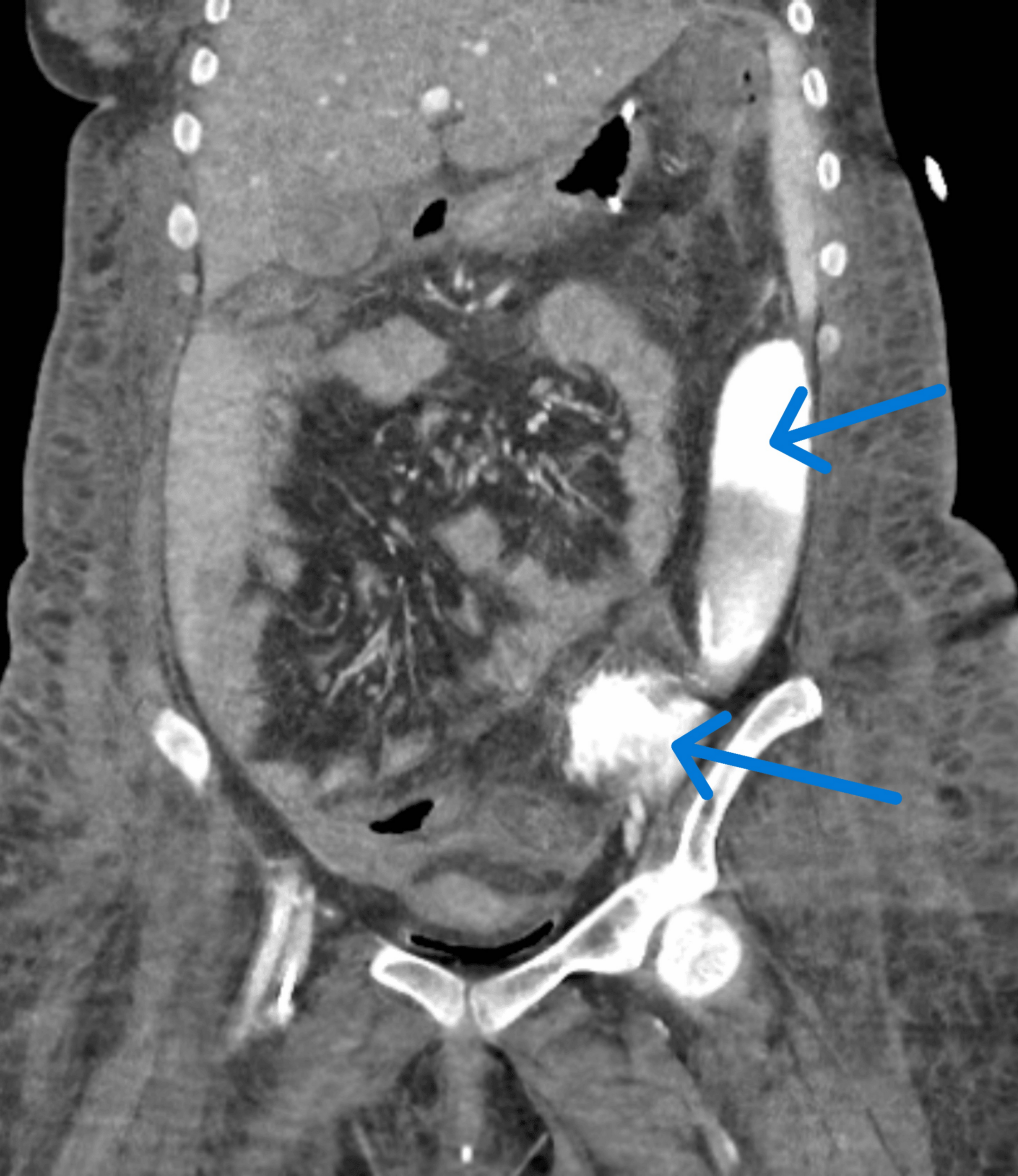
Contrast-enhanced CT of the abdomen and pelvis suspicious for a left distal ureteric calculus, resulting in the rupture/perforation of the left ureter in the left iliac fossa with resulting urinoma in the left retroperitoneal space, eventually extending into the peritoneal space in the left iliac fossa.
